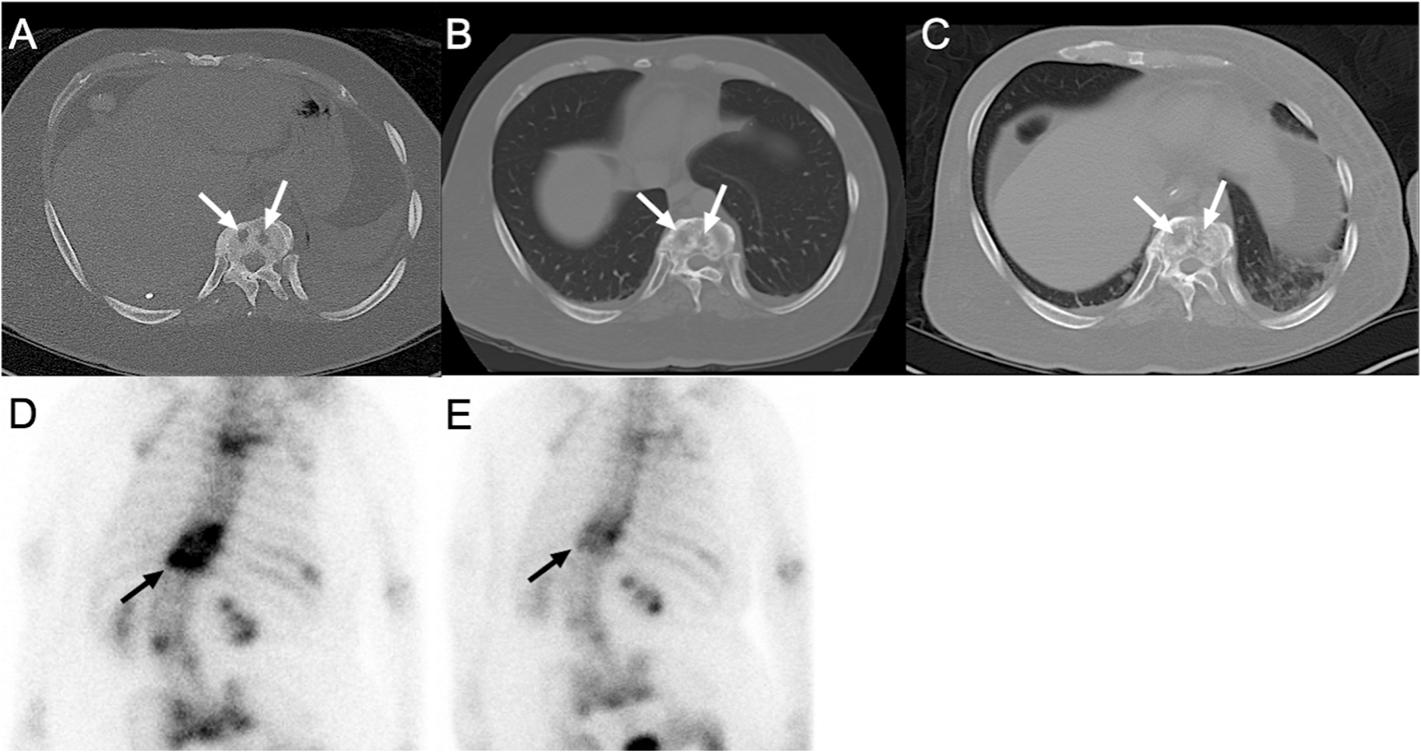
Bone density changes suggest tumor response in bone metastases. A 42-year-old man was diagnosed with lung adenocarcinoma and bone metastases. A Pretreatment axial CT scan in the bone window shows two osteolytic metastases (both lesions with diameters of 10 mm) (white arrows) in thoracic vertebrae. B, C The osseous lesions have not significantly changed in the sum of longest diameters according to RECIST 1.1 but show osteosclerotic reaction (white arrows) in 6 months (B) and 10 months (C) after targeted therapy with afatinib, an epidermal growth factor receptor (EGFR) - tyrosine kinase inhibitor (TKI), representing good response. D, E Skeletal scintigraphy shows significantly decreased uptake of radiotracer after comparison between pretreatment (D) and posttreatment (E) images, which confirmed good therapeutic response
